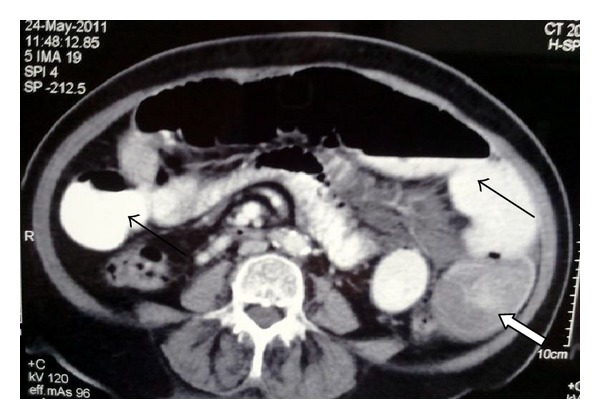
Computed tomography of abdomen showing dilated bowel loops (arrows) and the *target sign *of intussusception (white arrow).
